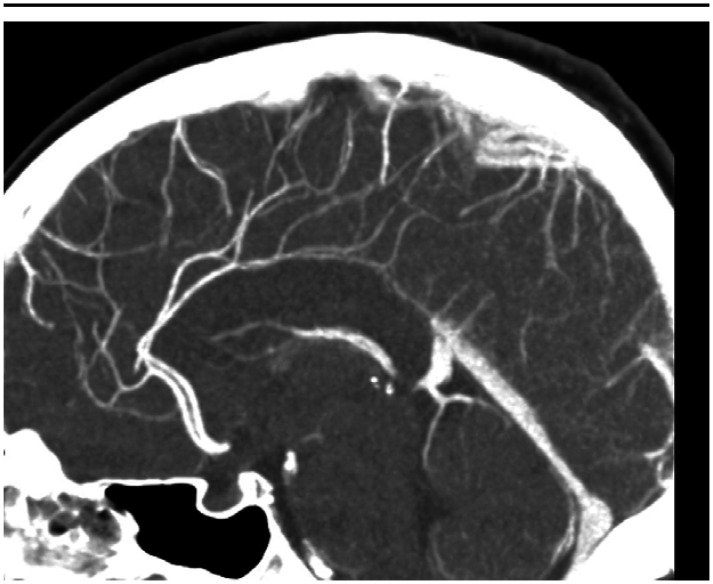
CTA showing normal cerebral arteries.
